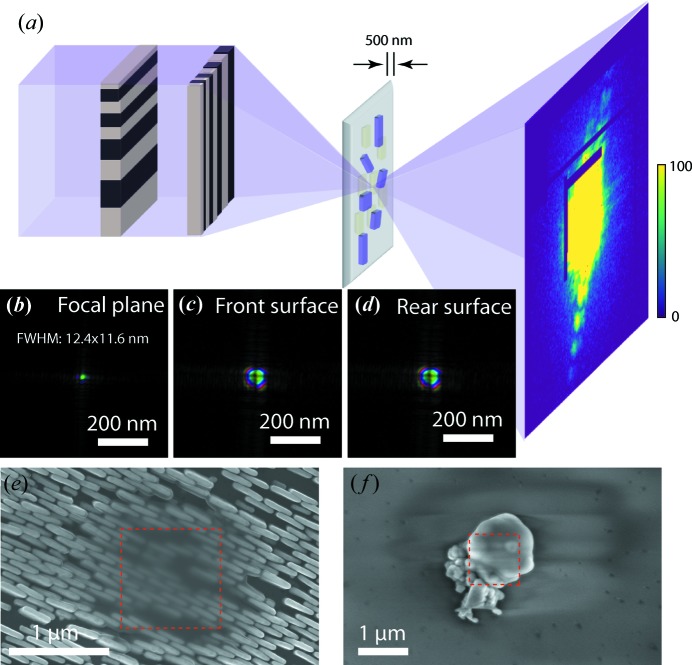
(*a*) Experimental setup for the multi-slice ptychography measurement. The order-sorting aperture (OSA) is not shown for simplicity. (*b*) The incident 12 keV X-ray beam was focused to a 12 × 12 nm spot by a crossed pair of MLLs. (*c*), (*d*) The X-ray wavefronts on the front and rear surfaces of the sample, which was placed 10 µm downstream from the focal plane. (*e*), (*f*) The SEM images of the gold zone plate structure and nickel oxide particles on the front and rear surfaces measured with 30 and 5 keV electron-beam energies, respectively. The dotted red boxes indicate the X-ray scanned area.
